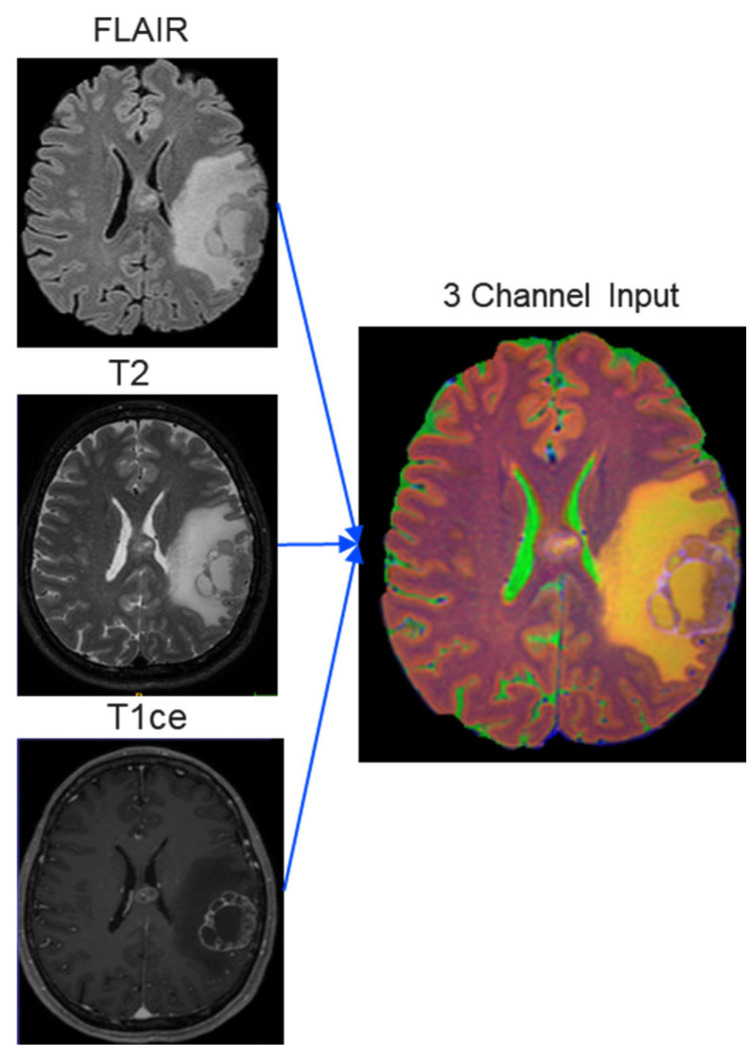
The three MRI modalities registered and combined to act as inputs to U-net model.
